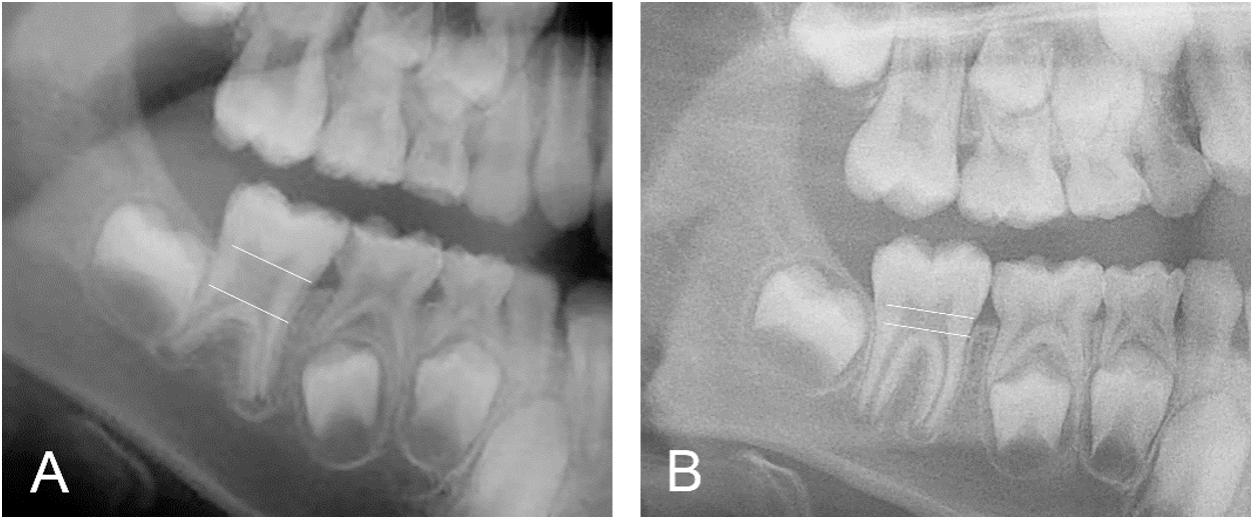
Radiographic appearance of taurodontism. Two eight-year-old boys with OI type I, quantitative defects caused by a mutation in *COL1A1* and no DGI. A. In this individual, apically extended pulp chamber, taurodontism, in the right first permanent lower molar is seen. B. Normal morphology of the corresponding tooth in the other boy.
